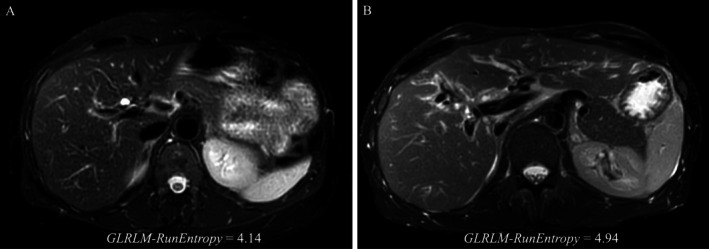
Two FS‐T2W sequences analysed in this study. Patient A is a low‐risk patient (MRS < 0, alkaline phosphatase within normal range, LSM 6.5 kPa, stable disease over time; *GLRLM‐Run Entropy* 4.14). Patient B exhibits intense fibro‐inflammatory activity (alkaline phosphatase 3.4×ULN, LSM 15.3 kPa) and underwent liver transplantation 20 months post‐MRI due to cholangiocarcinoma onset (*GLRLM‐Run Entropy* 4.94). The differences appreciable to the human eye are minimal but are quantitatively reflected in the radiomic feature.
